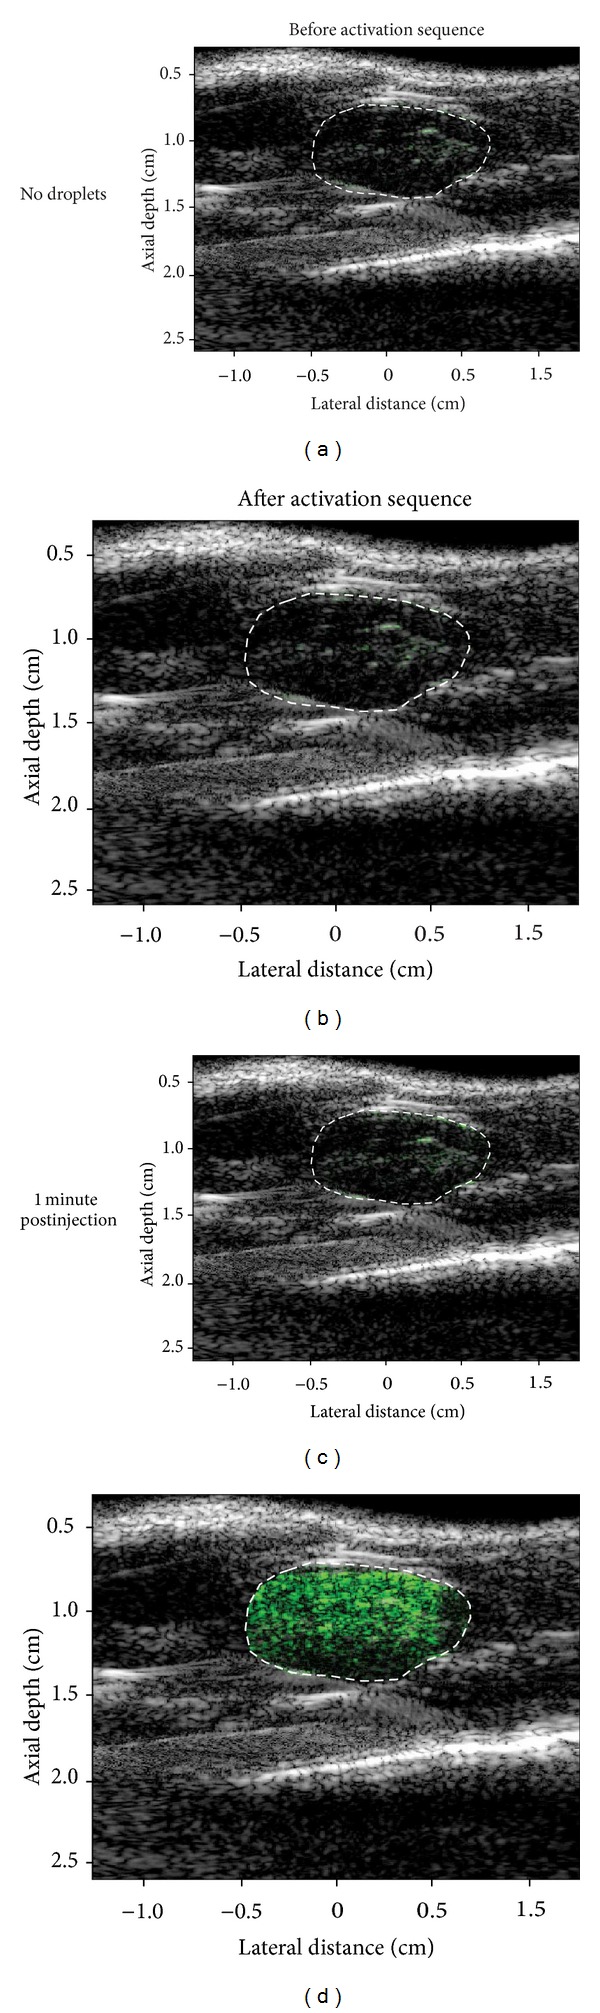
Overlays of contrast-specific intensity (green scale) and traditional B-mode (grey scale) ultrasound scans within the region of interest (white dotted line). When no droplets are present (top row), very little contrast is present before (left) and after (right) the vaporization pulse sequence. When a bolus of OFP droplets is injected, no significant increase in contrast is observed before the activation sequence (bottom row, left), but once the activation sequence is delivered a high level of contrast is visible in the region of the kidney (bottom row, right)—indicating conversion of the droplets to form highly echogenic microbubbles. Unpublished data.
